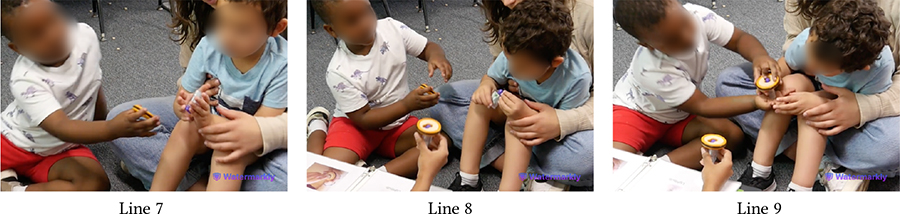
Table 14: T14: Powerful ideas of CT [6, 54] learned by neurodivergent children and factors that impacted their learning.
